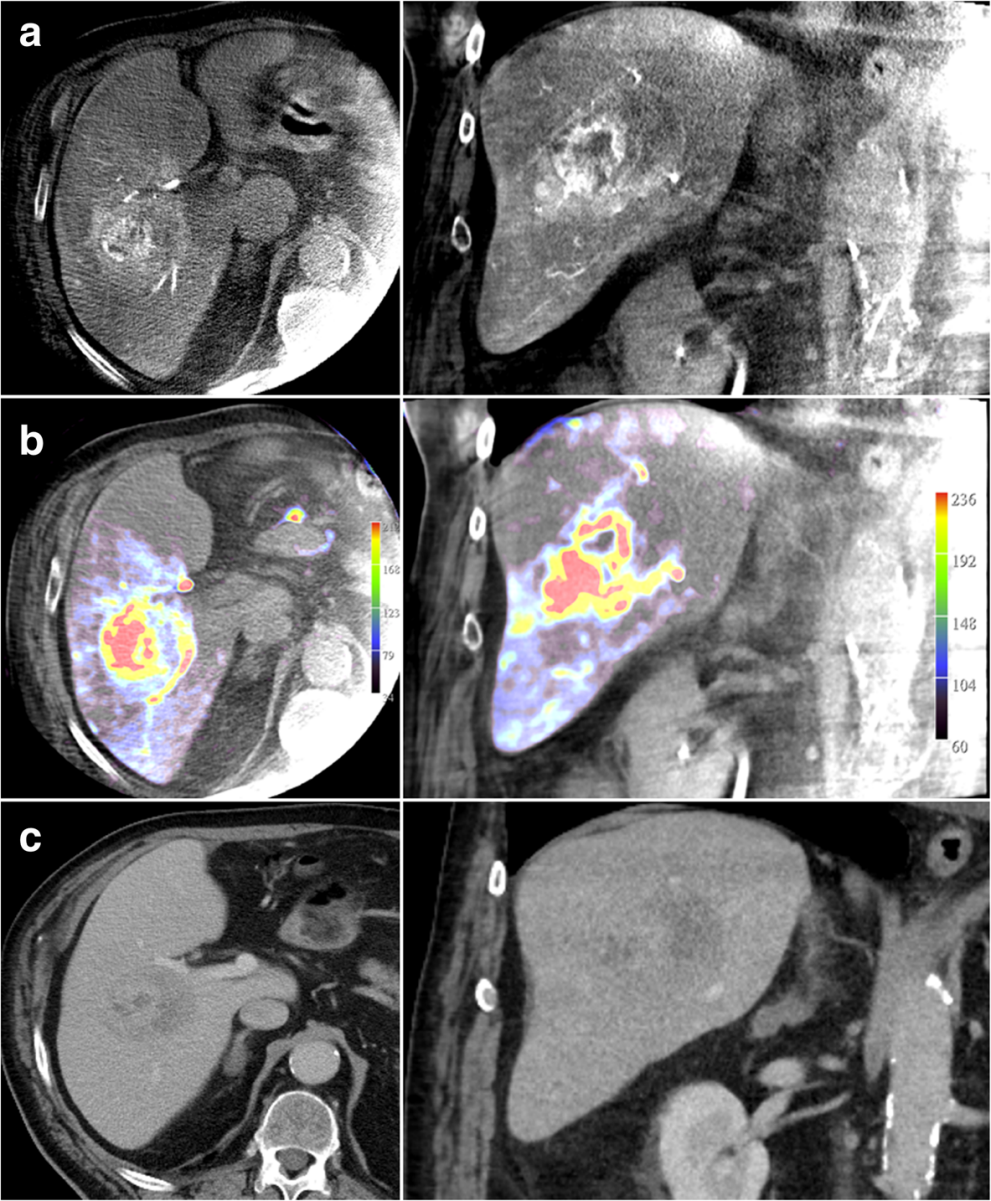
Images of HCC lesion at liver segment V of a 82-year old male patient in arterial phase (a), PBV map (b) as well as pre-interventional CT at portal venous phase (c)
